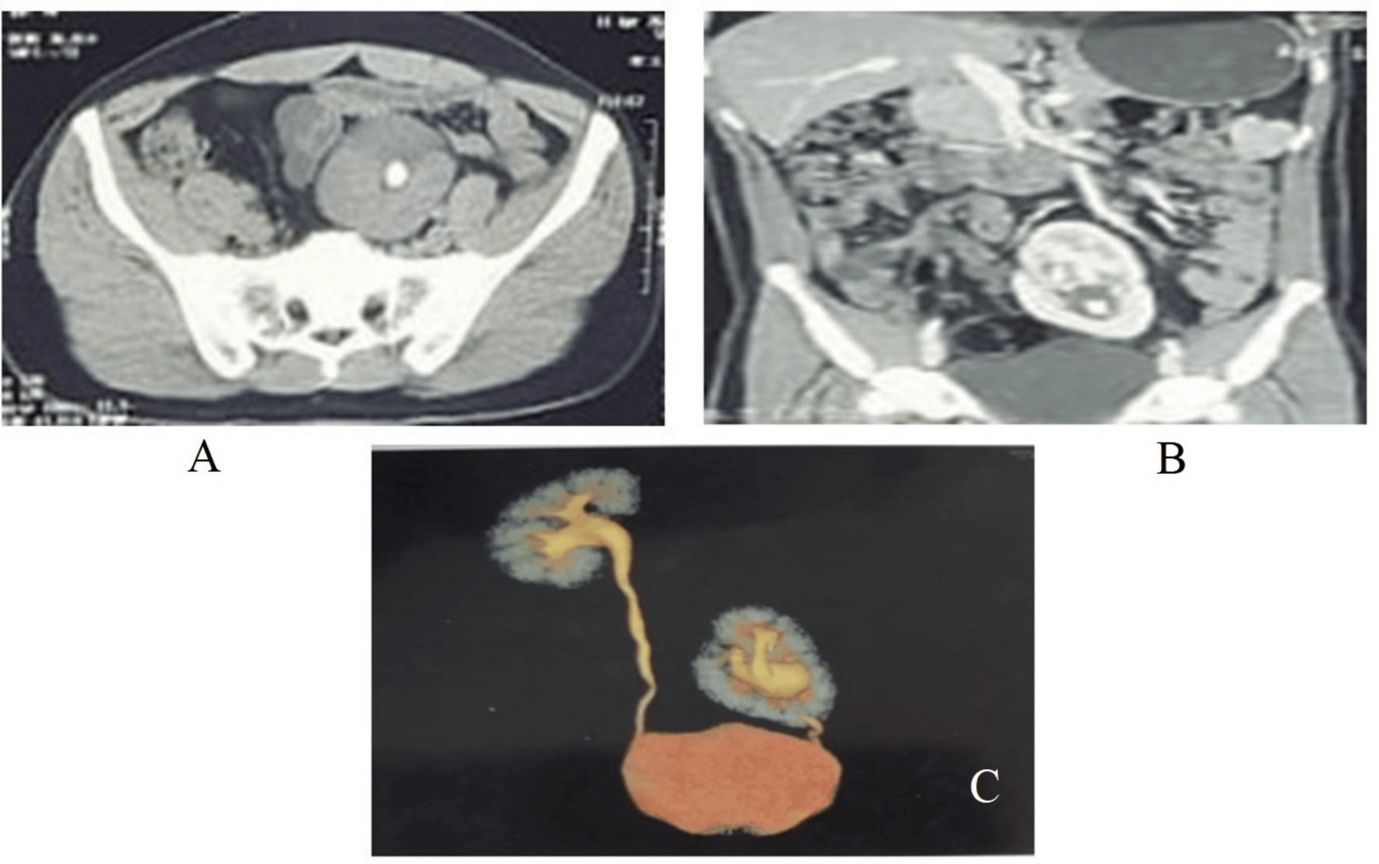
(A) Preoperative CECT KUB in a patient with a single large stone in an ectopic left-sided kidney and (B) shows urography. CECT KUB, contrast-enhanced computed tomography of the kidneys, ureters, and bladder
